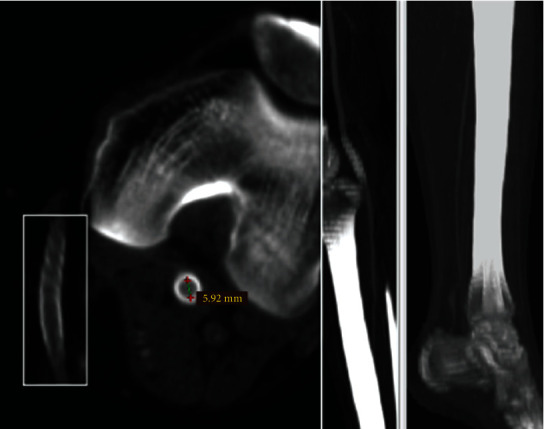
Follow-up CT angiography: complete exclusion of the aneurism and preserved patency of the posterior tibial artery.
